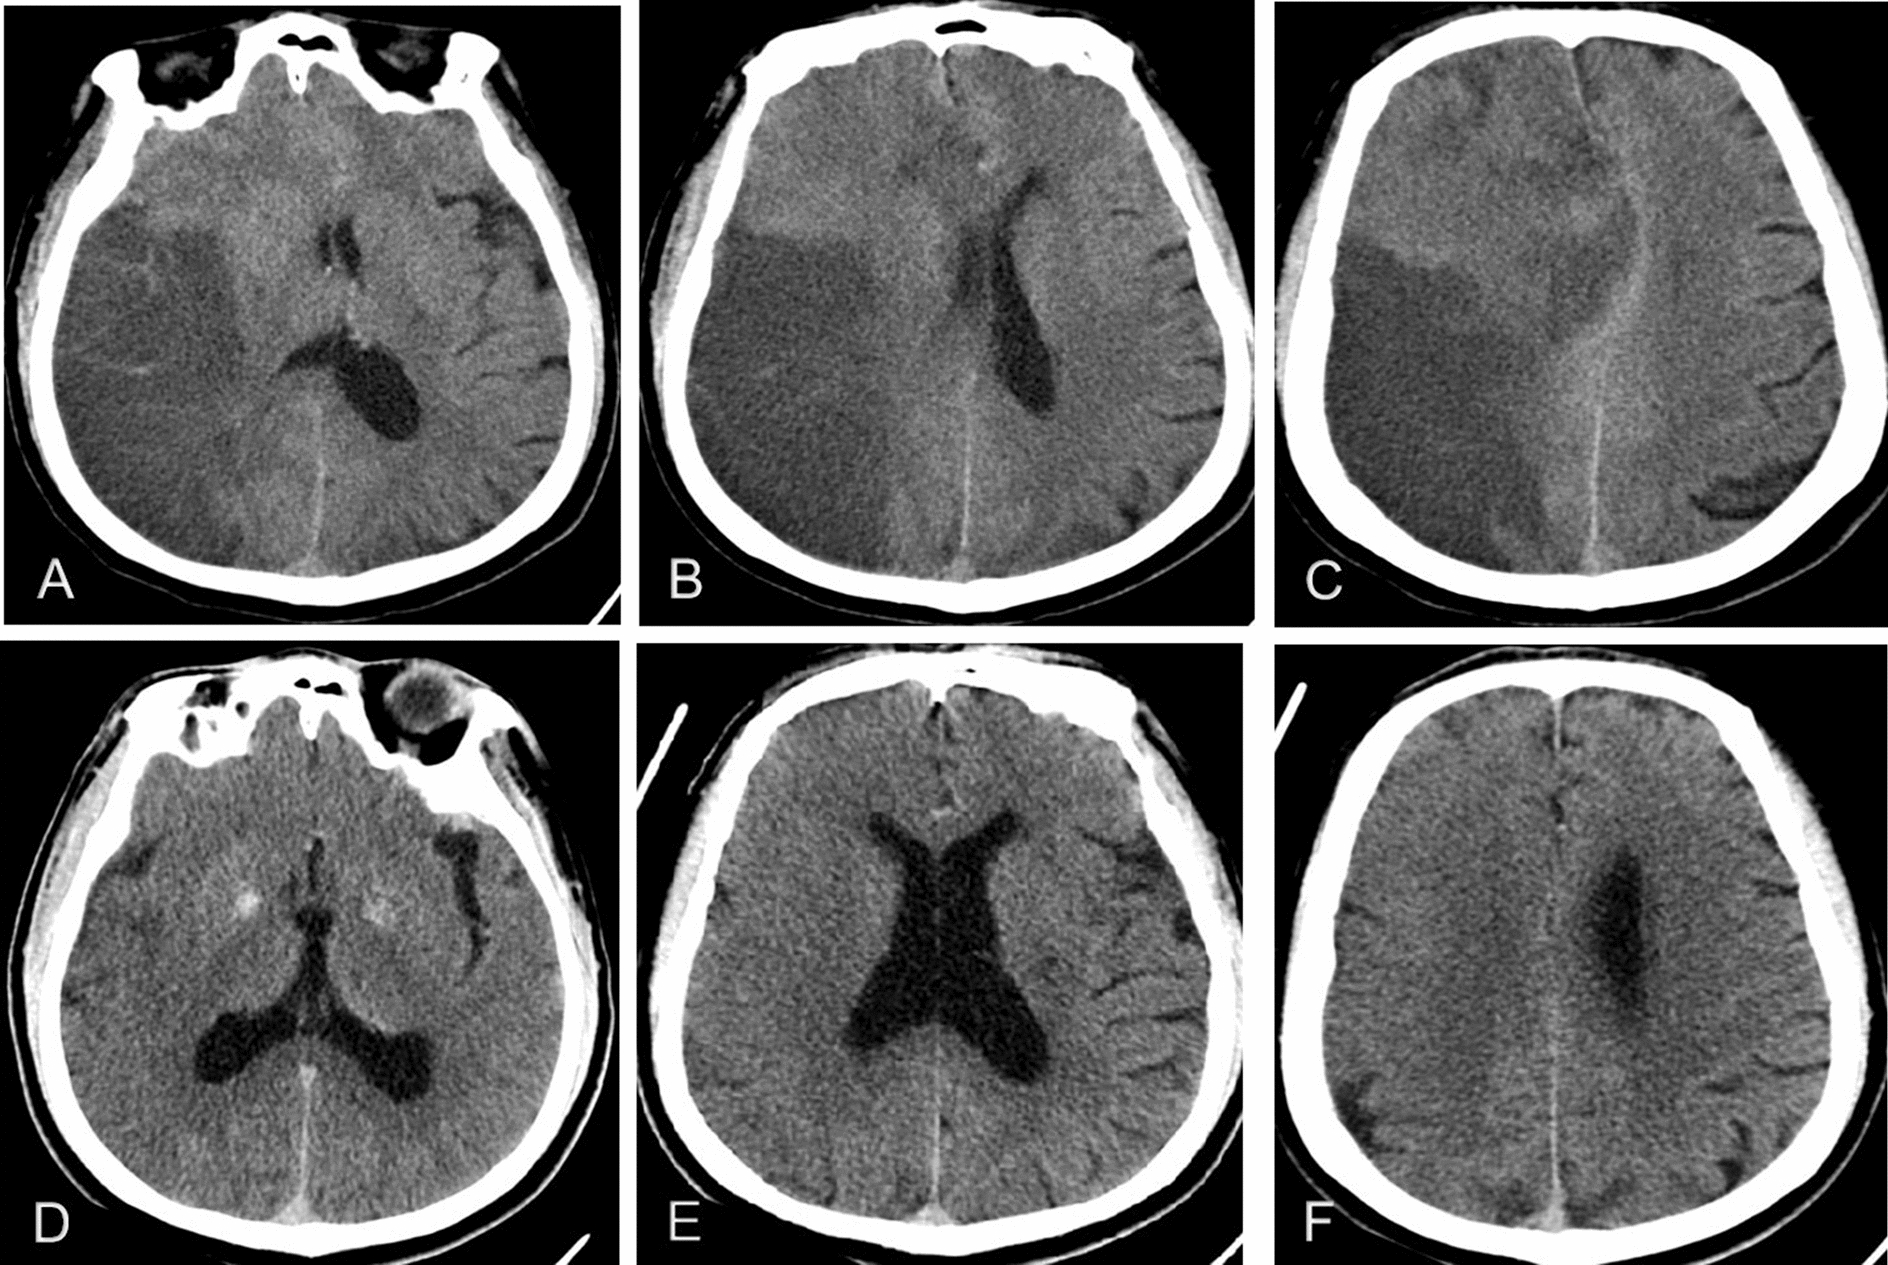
Typical images of MBE after MT: 59-year-old man with mTICI score, 2b; and mRS score, 4; A–C post-treatment patient with cerebral edema on CT image; D–F pre-treatment patient without cerebral edema on CT image
